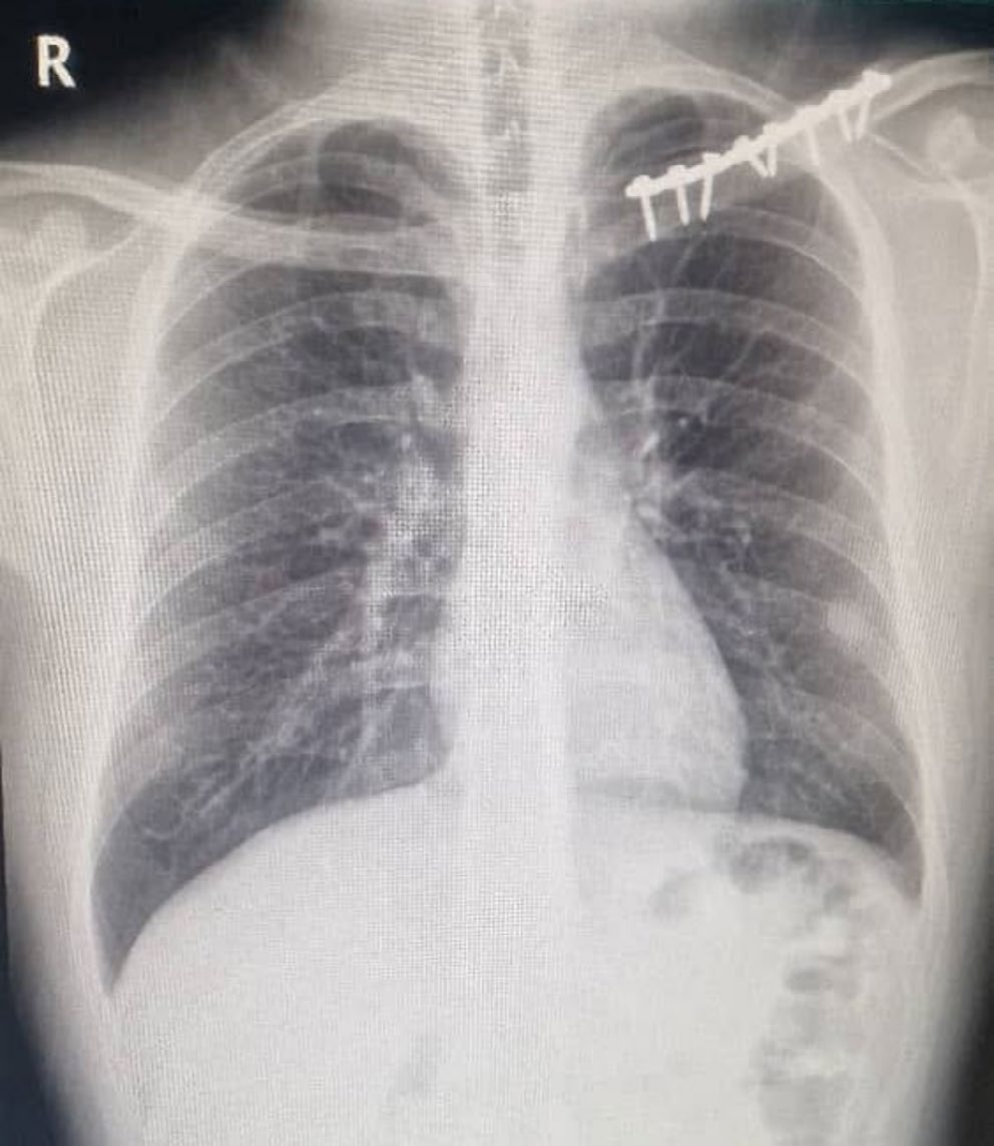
Posteroanterior chest radiograph showing left clavicular fixation hardware with otherwise clear lung fields and a subtle pleural‐based opacity along the lateral aspect of the left lower hemithorax.
